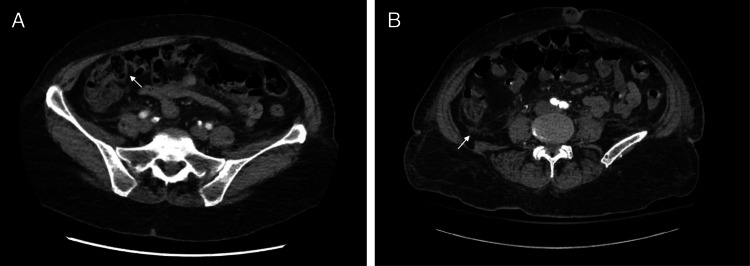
Initial CT abdomen/pelvis with contrast (A) Wall thickening in the ascending colon and terminal ileum, suggestive of ascending colitis. This territory is supplied by superior mesenteric artery. (B) Surrounding fat stranding, which may indicate inflammation or ischemic insult.
